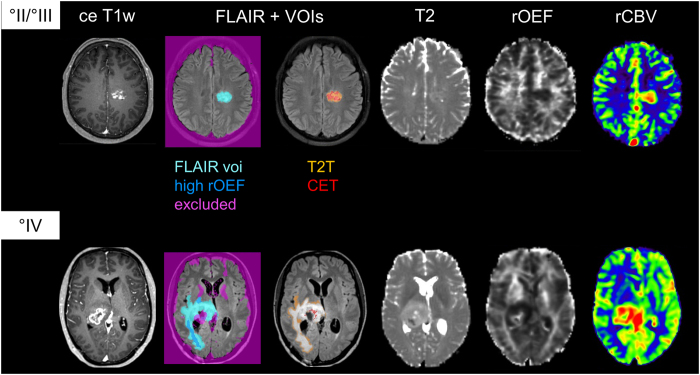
Examples of a WHO grade II/III glioma (top row) and WHO grade IV glioblastoma (bottom row) and VOI definition. Sequences shown are contrast-enhanced T1w, FLAIR, T2, rOEF and CBV.
